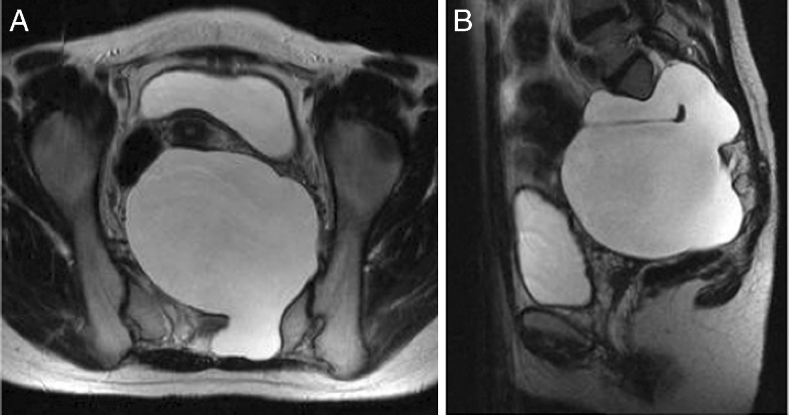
a, b T2-weighted MRI in case 10 revealing a huge presacral mass (11 × 10 × 9 cm). The huge sacral meningeal huge enters the presacral space through the left S2 and S3 foramina
